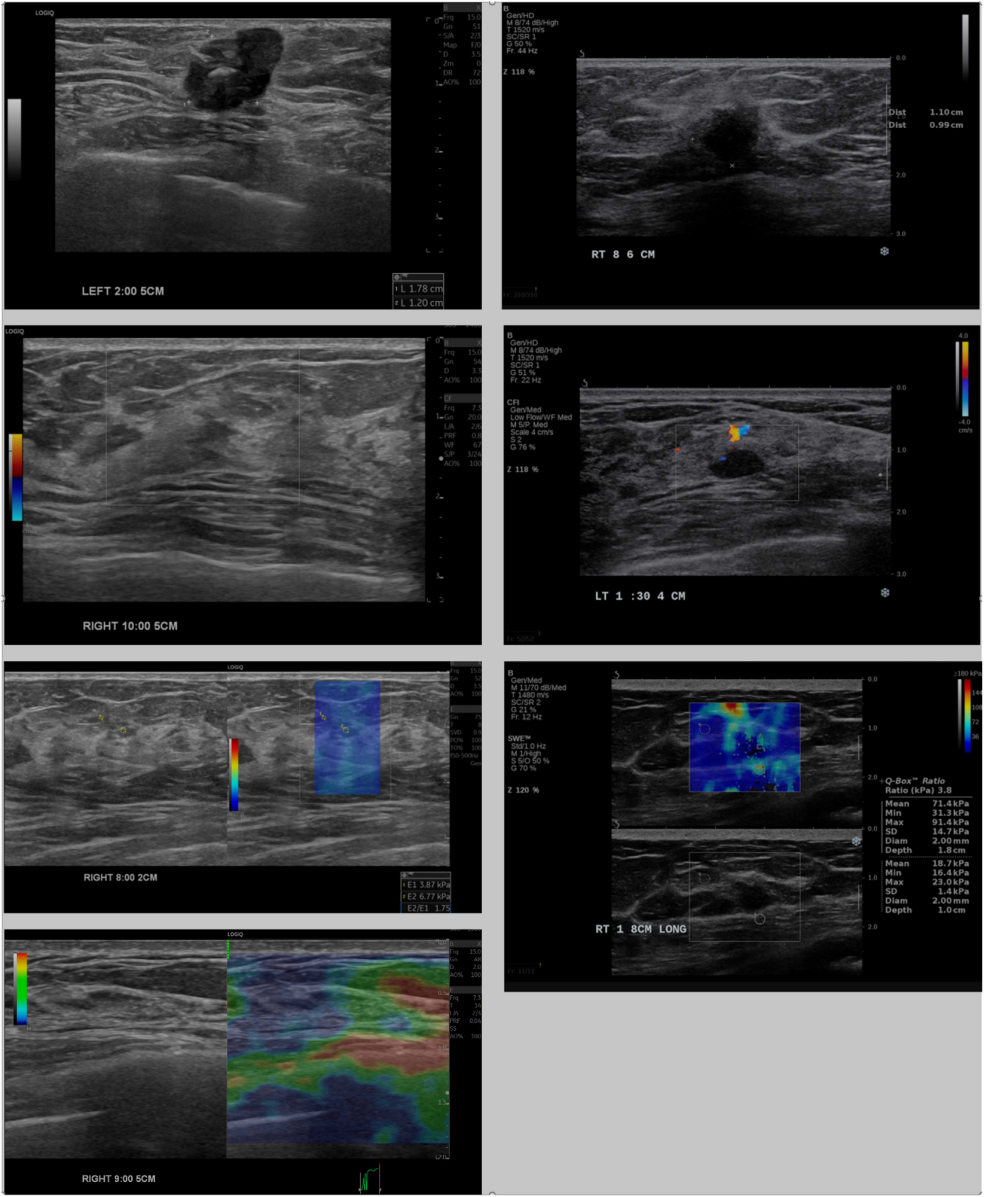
Comparison of breast ultrasound images acquired from LOGIQ E10 (left) and Aixplorer (right). Rows show, from top to bottom: B-mode images with annotated mass sizes, Doppler images, shear-wave elastography, and strain elastography (available only for LOGIQ E10).
